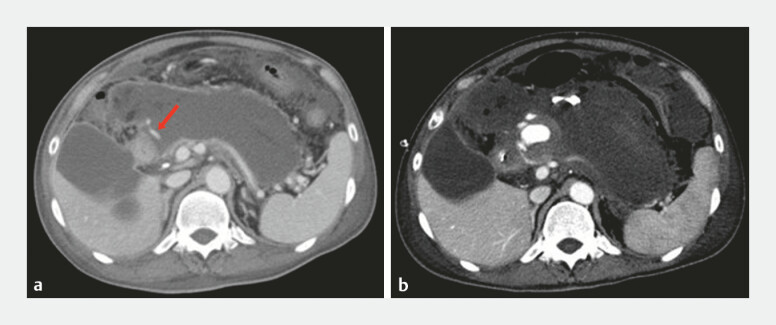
Computed tomography images showing:
